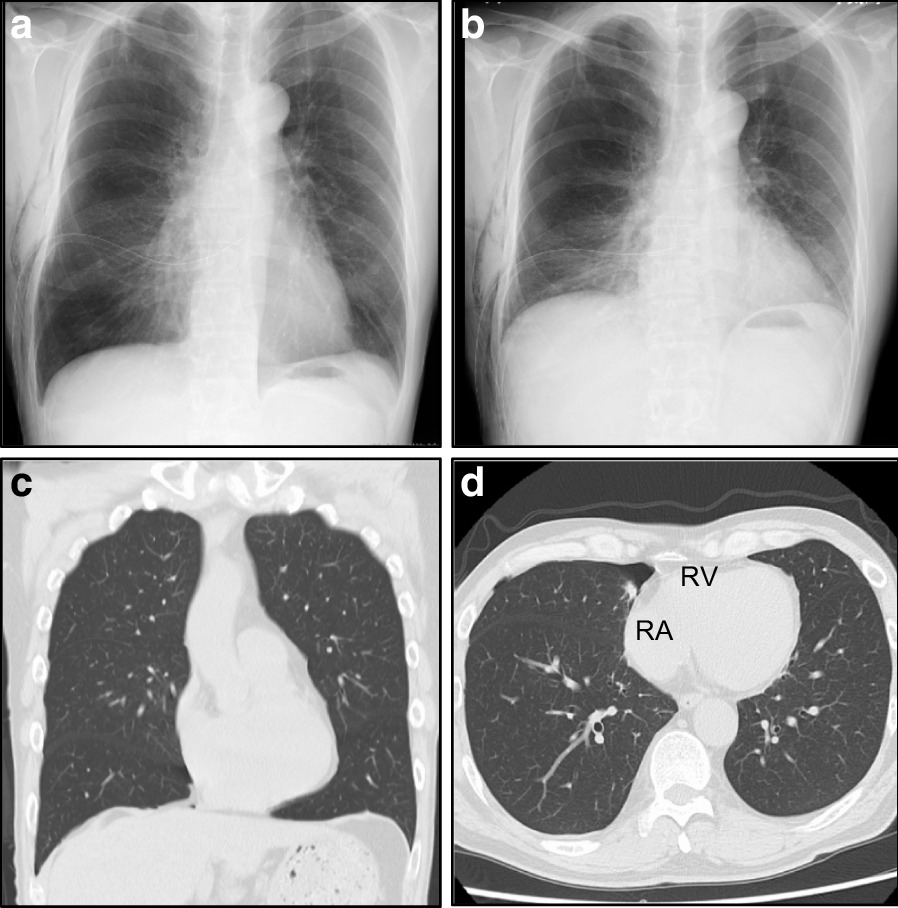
Follow-up chest radiographs and computed tomography scans of the post-tube thoracostomy (a, c, d: during inspiration, b: during expiration). a–d Follow-up chest radiographs and computed tomography scans reveal the resolution of pneumothorax. The resolution of right atrial collapse can be noted. *RA* right atrium, *RV* right ventricle
